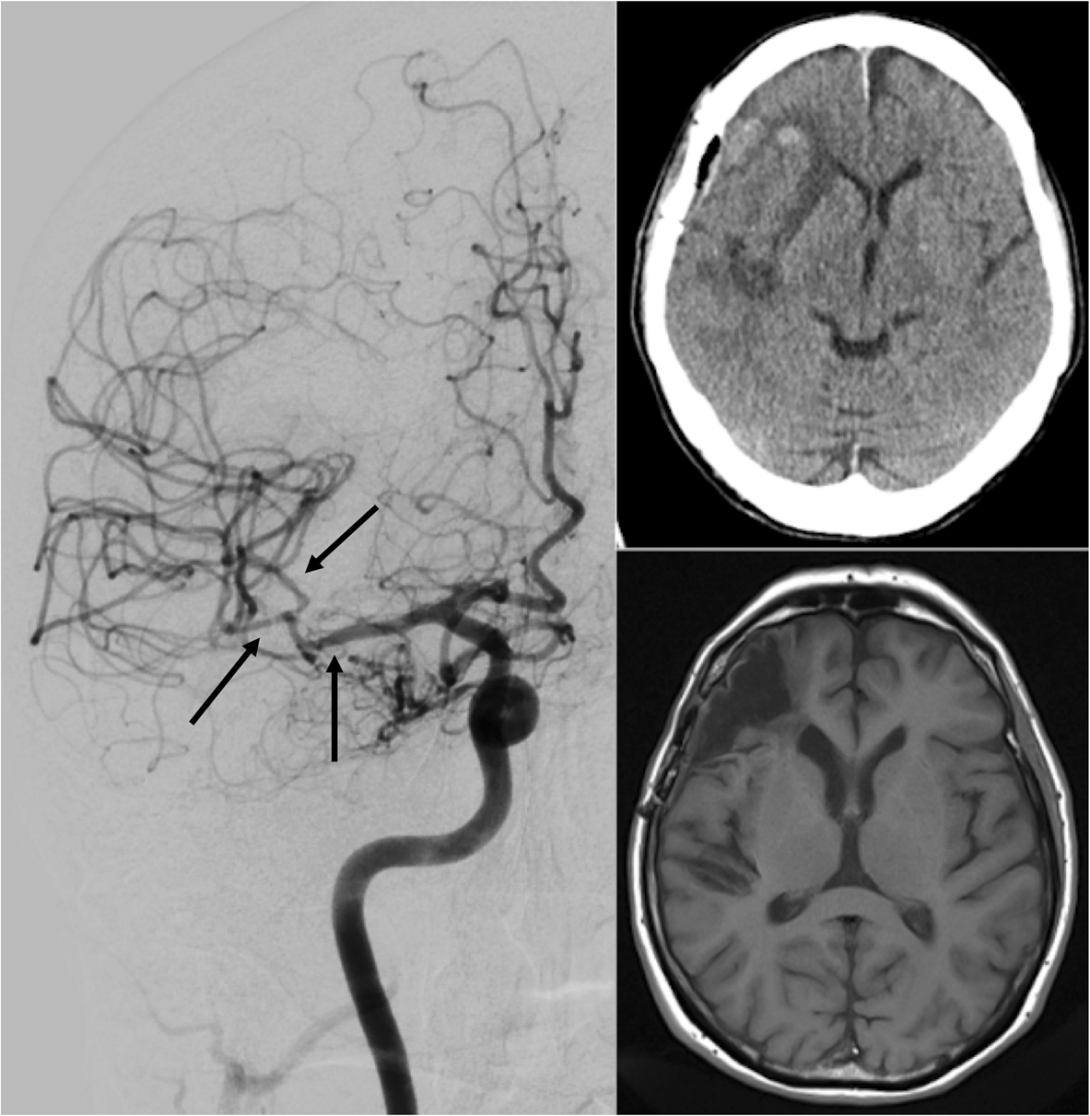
Postoperative imaging with proof of vasospasm of the M1 and M2 segments of the right MCA (black arrows), CT imaging at admission and MRI follow up after 6 months
